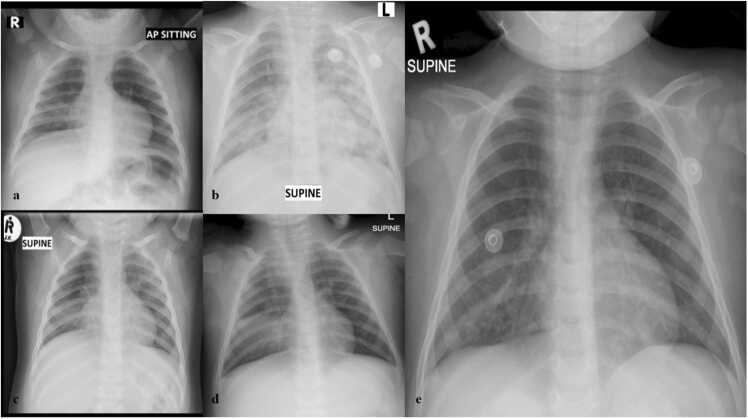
Chest X-ray findings: a) Chest x-ray at presentation: Right middle lobe changes present. b) Develops bilateral infiltrates in all the zones. c) X-rays showed improvement with the right middle lobe involved. d) Chest x-ray at PICU admission, shows progression of infiltrates again and consolidation in right middle lobe progressing. e) Chest x-ray at discharge from PICU shows significant clearing with mild right middle lobe changes.
